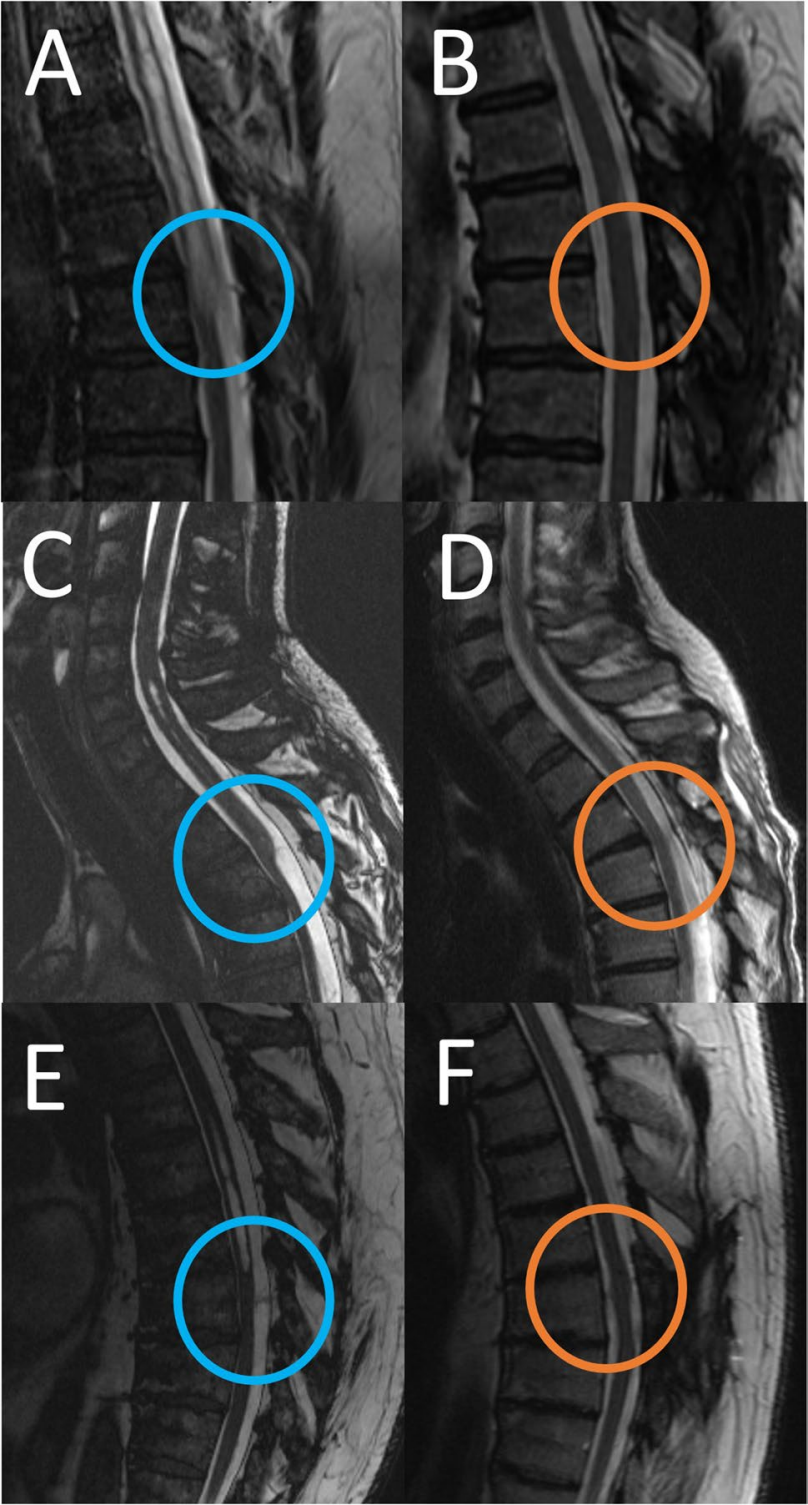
Preoperative (A, C ,E) and postoperative (B, D, E) sagittal MRI of the three patients with SAW. The preoperative caliber variation (blue circle) improved 3 months after surgery (orange circle)
